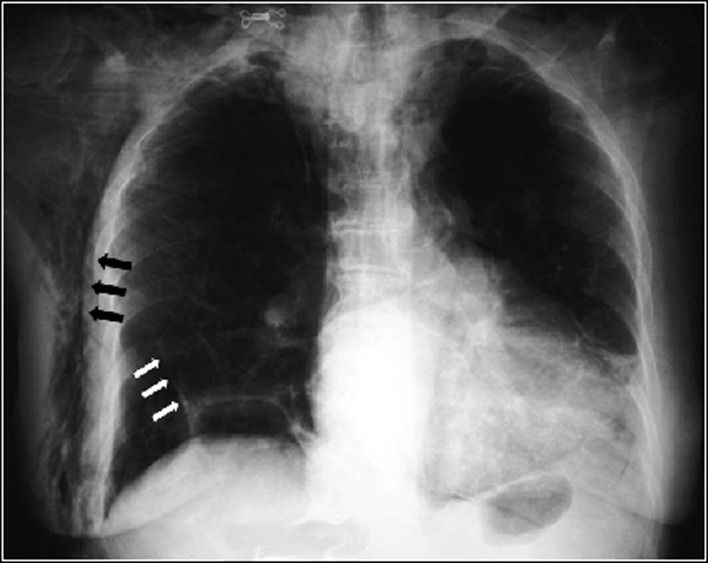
Chest x-ray showing diffuse subcutaneous emphysema (black arrows) and a right-side pneumothorax (white arrows).
